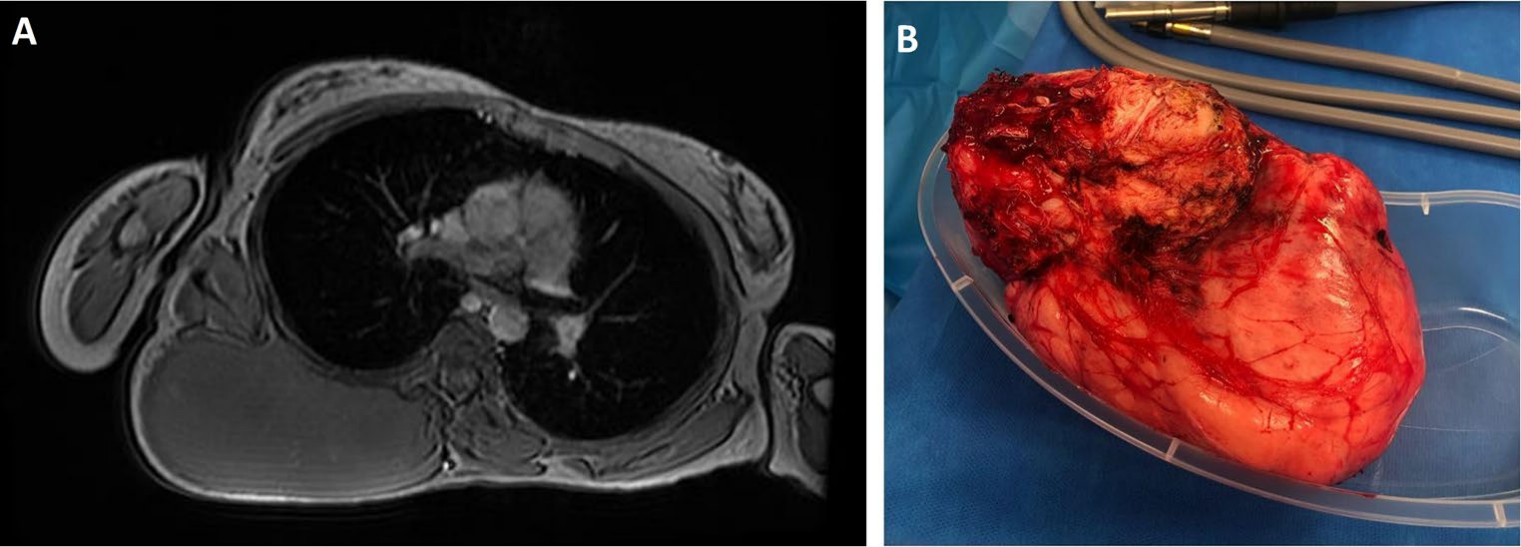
A: MRI axial image showing extra-abdominal desmoid - located in the thoracic paraspinal location. B: Photo of the desmoid once removed
